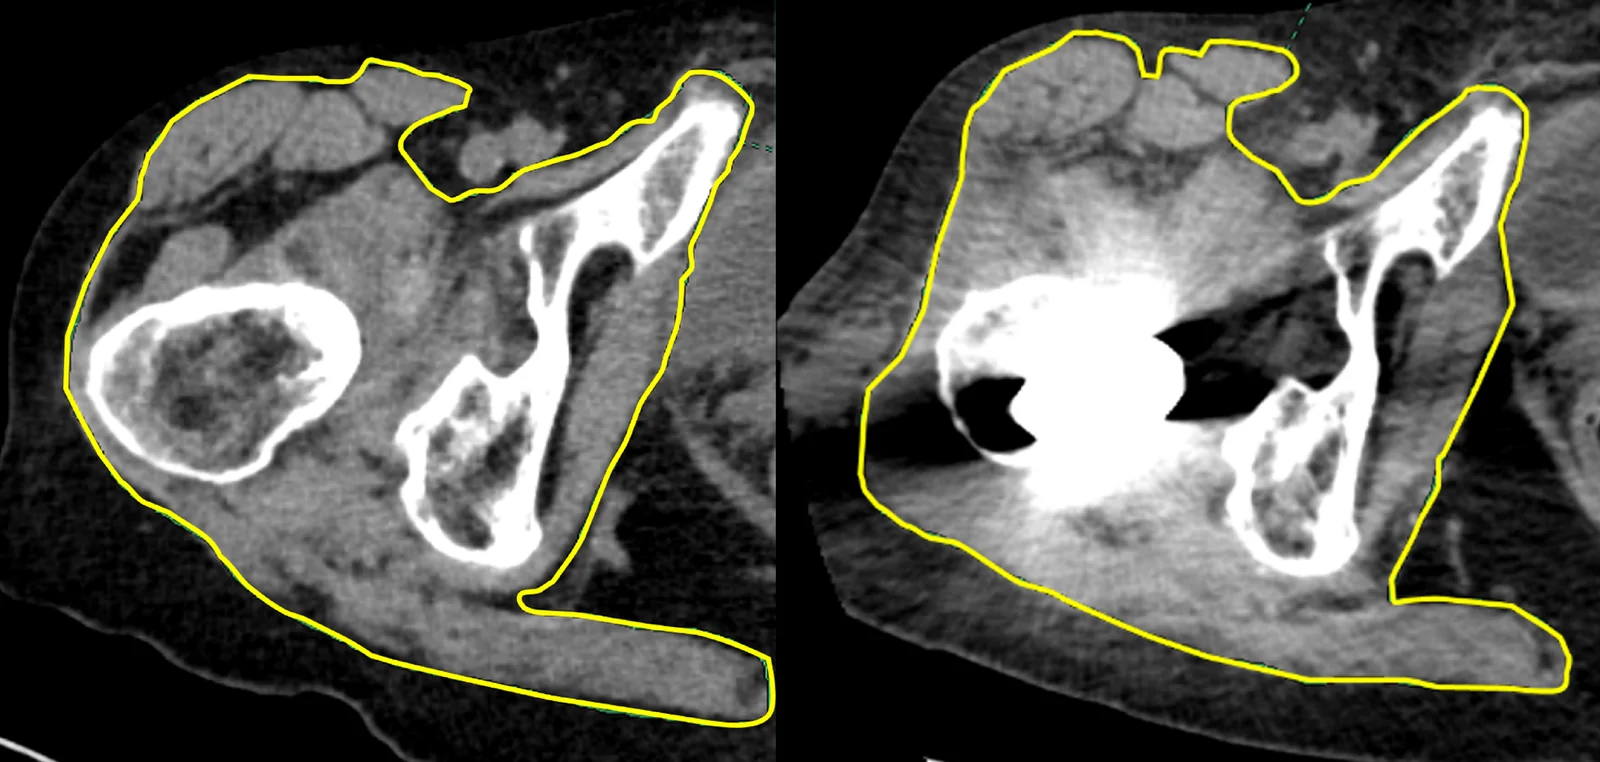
Preoperative (left) and postoperative (right) axial computed tomography images at the teardrop level, showing the under-fascial area outlined in yellow
